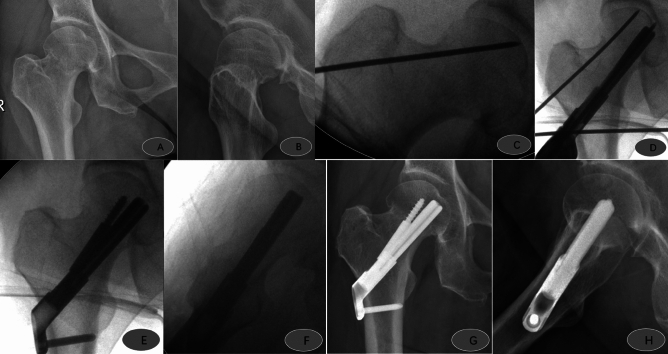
Male, 24 years old, admitted to the hospital with right hip pain and limited movement due to a fall; preoperative anteroposterior (A) and lateral (B) views showed right femoral neck fracture, Garden I, type 1; in situ fixation with FNS was placed 2 days after the injury; intraoperative operations are shown in Figures (C, D, E and F). Postoperative anteroposterior (G) and lateral (H) radiographs 3 months after surgery showed good fracture healing and satisfactory internal fixation position.
